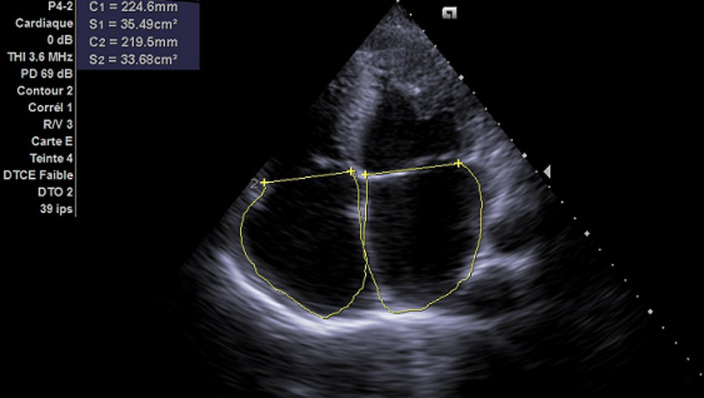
two-dimensional transthoracic echocardiography in apical 4-chamber view finding a noticeable reduction of left ventricular volume by a thrombotic materiel with calcification which also affects the mitral subvalvular apparatus
